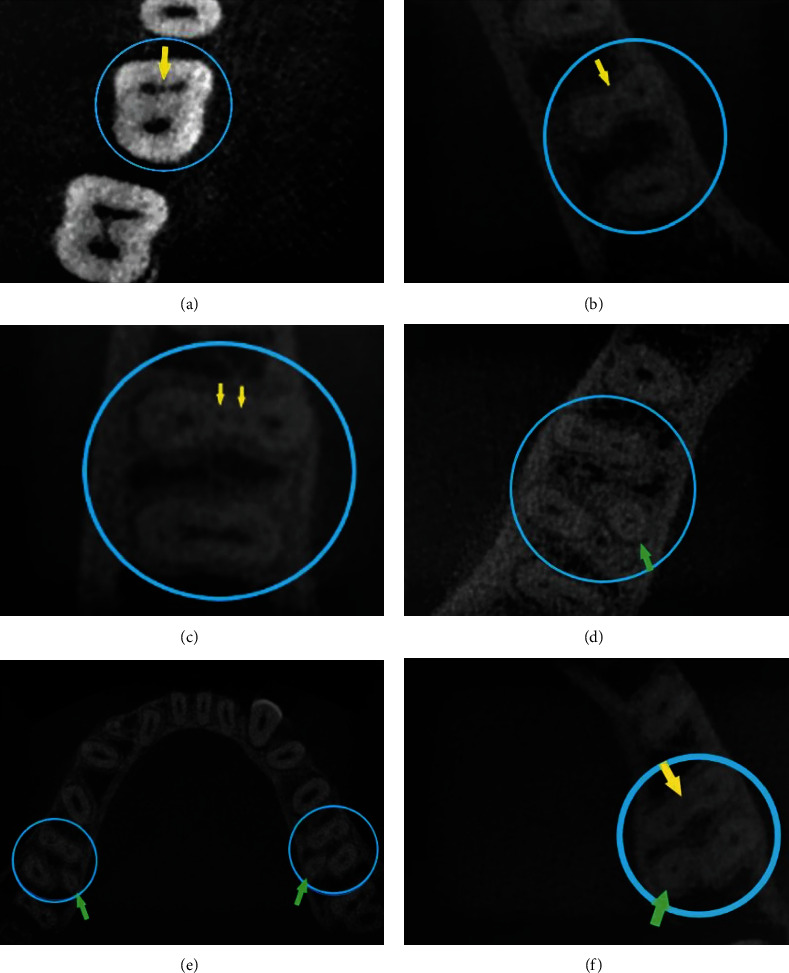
Cases of mandibular first molars with MMC or RE in the axial sections: the blue circles indicate the examined teeth; the yellow arrows indicate MMCs; the green arrows indicate REs. CBCT images (a–c) show MMCs, (d–f) RE, (e) bilateral RE, and (f) MMC with RE.
